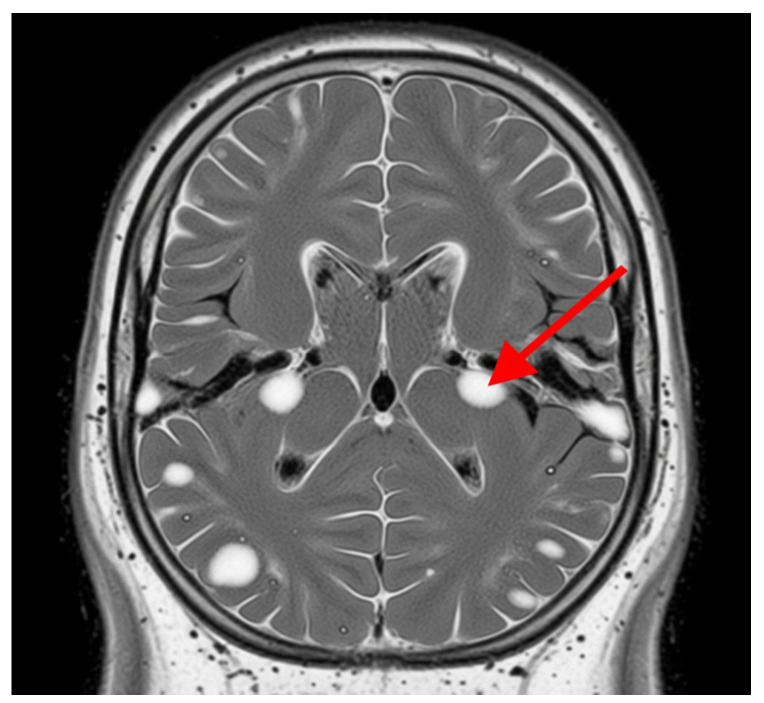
White matter lesions. The grey area represents the brain tissue, and the bright white areas represent the white matter lesions. These lesions are typically hyperintense (brighter) on T2-weighted MRI scans and represent areas of damage to the myelin sheath, which insulates nerve fibers in white matter. The red arrow indicates one of these distinct bright white lesions. In SPMS, these lesions indicate demyelination, axonal loss, and gliosis.
